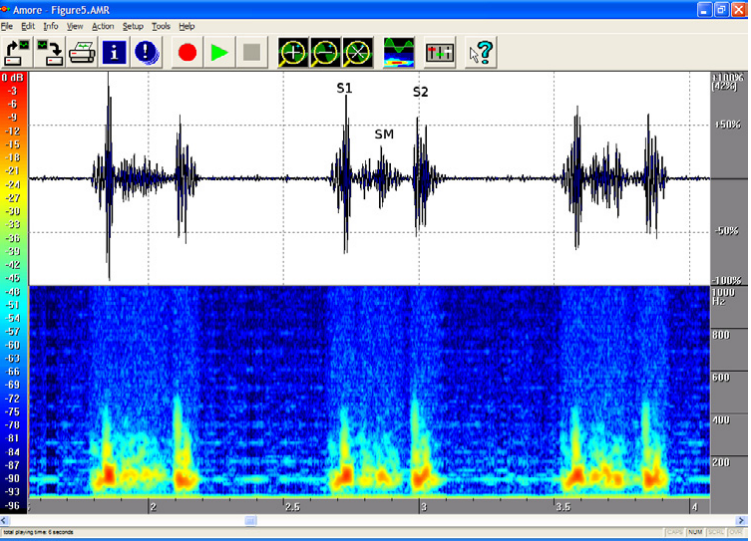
Pulmonary stenosis in a 9-year-old. Haemodynamically insignificant pulmonary stenosis, with a 17–22 mmHg flow gradient on echocardiography. On auscultation a second-degree coarse, low-frequency systolic ejection murmur was heard. The phonogram shows the diamond shape compatible with stenotic murmur. Flow velocity is highest at the beginning of systole, and the peak frequencies also occur in early systole, with a descending frequency contour. The findings resemble ASD, the main difference being that P2 is quieter than normal. Because the stenosis is mild, however, P2 can be heard. Area of auscultation: left second ic space (LIC2). Duration 90%, peak frequency 264 Hz, volume 52 %.
